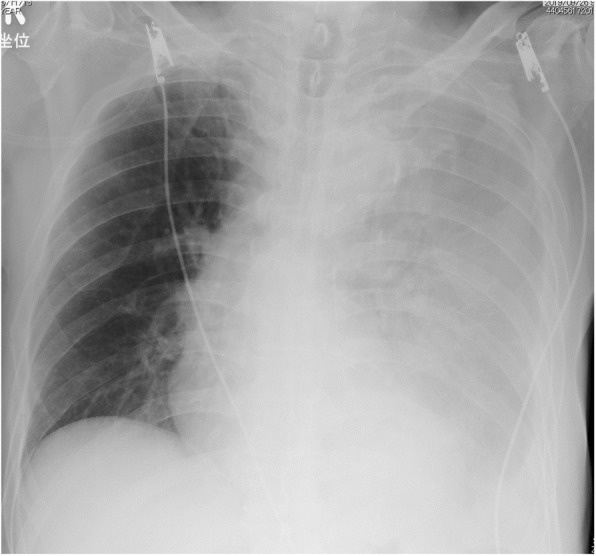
Chest X-ray showing decreased permeability of the left lung field
